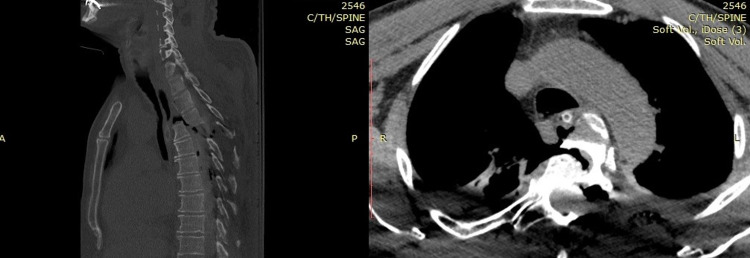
Computed tomography of the thoracic spine Sagittal CT reconstruction of the thoracic spine demonstrating posterior dislocation of T3-T4.
